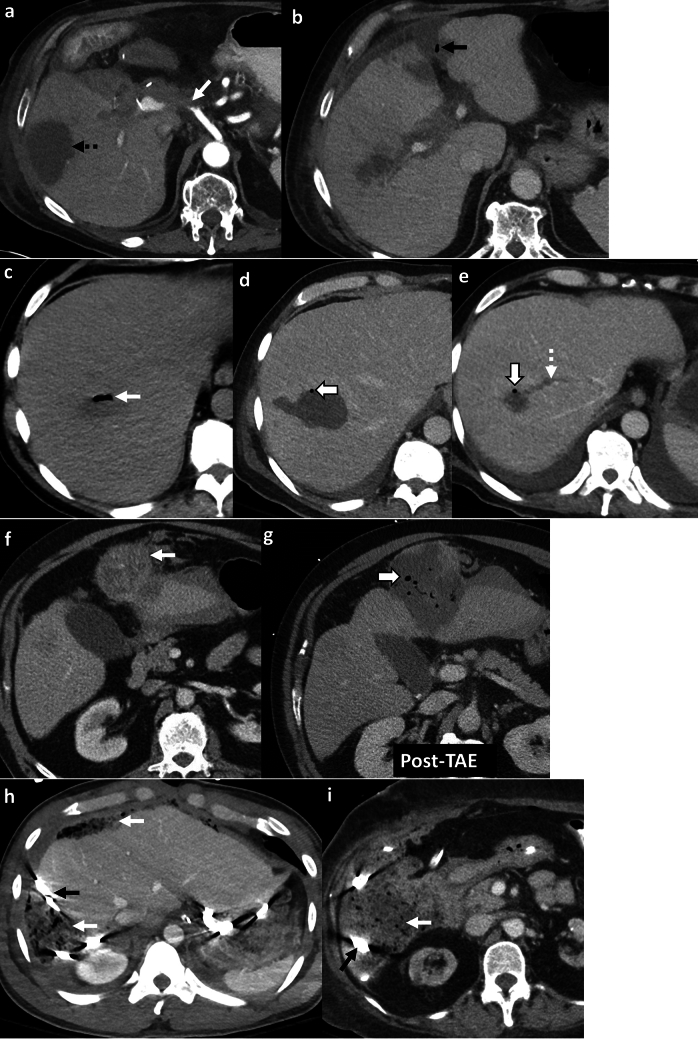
Pitfalls for emphysematous hepatitis. a, b Infected biloma: A 60-year-old post-Whipple’s procedure with fever and abnormal liver function tests. CT images show a thrombosed hepatic artery (solid white arrow, a), multiple bilomas (dotted black arrow, a), some with air (solid black arrow, b). c–e Post-ablation changes with infection: A 48-year-old post-Whipple’s procedure and hepatic metastasis ablation. Initial non-contrast CT (c) shows expected post-procedural air within the ablated region (solid white arrow, a). Follow-up CT at 3 weeks d, e shows increased size of ablated lesion with air locules (block white arrows, e, f) and a middle hepatic vein thrombus (dotted white arrow, e) extending to the inferior vena cava (not shown). A rapid increase in the size, increased fluid component, and sepsis were suggestive of hepatic abscess, which was confirmed on aspiration. f, g Post-embolization changes: A 63-year-old with hepatic cirrhosis and bleeding hepatocellular carcinoma (solid white arrow, f) treated with trans-arterial bland embolization (TAE). Post-TAE CT two days after embolization g shows expected post-procedural air foci in the treated lesion (block white arrow, g) with decreased enhancement due to tumor necrosis. Findings resolved on follow-up, and patient’s serial WBC counts were within normal limits. h, i Post-laparotomy sponges: A 60-year-old post-polytrauma damage control surgery with laparotomy sponges in perihepatic region and gallbladder fossa (solid white arrows h, i). Note radio-opaque ribbon markers (black arrows, h, i) indicating sponges
